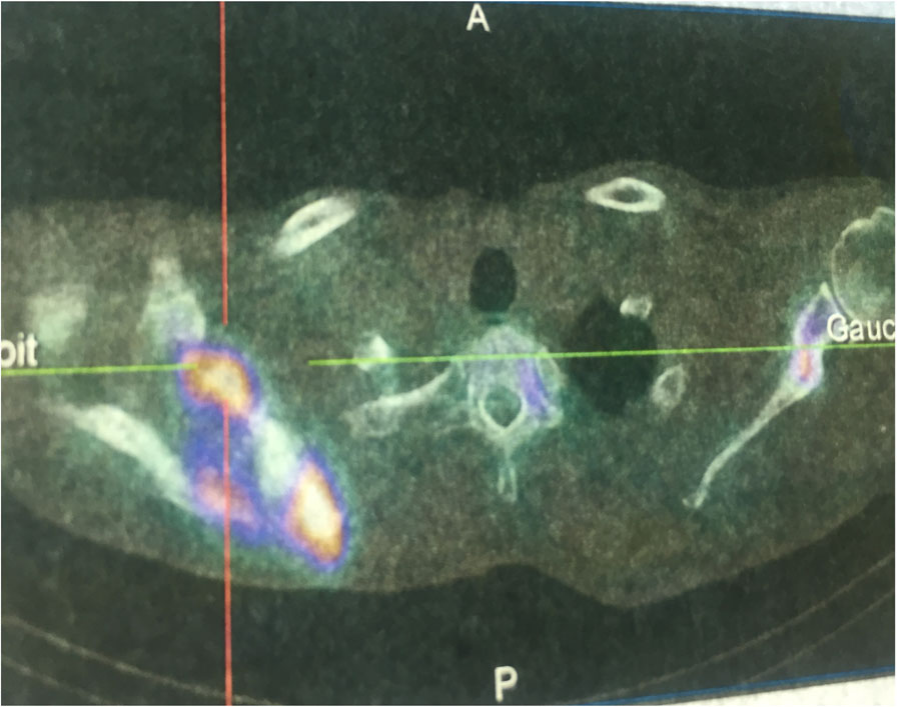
Single-photon emission computed tomography-computed tomography with osteocondensing and lytic aspect in the spine of the right scapula
